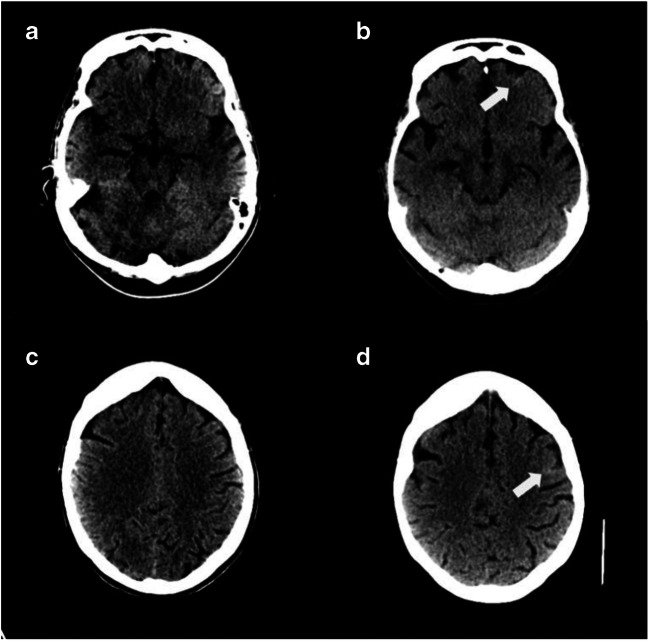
Non-aneurysmal SAH co-existent with COVID-19 (non-contrast CT). Contemporary non-contrast CT brain imaging for patient B was compared with that acquired 8 years previously and demonstrated multifocal, curvilinear foci of sulcal high density consistent with SAH (non-contrast CT head from 2011 a and c, 2020 b and d with arrows highlighting areas of high density)
